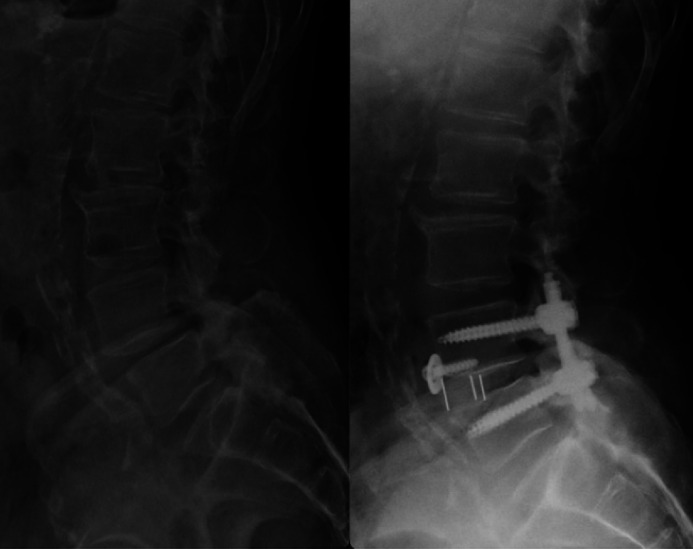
Pre and postoperative lateral X-ray of a patient with AAC that underwent MIS-ATP lumbosacral fusion.
